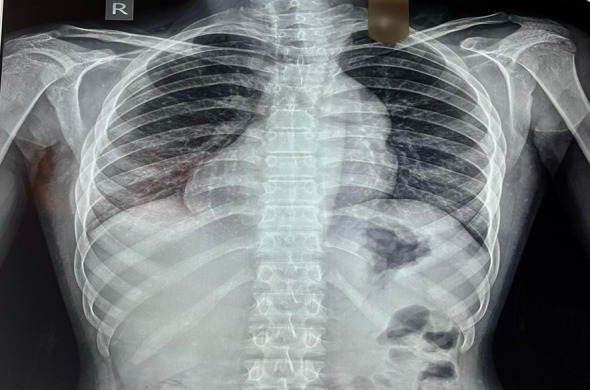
Echocardiography findings are
